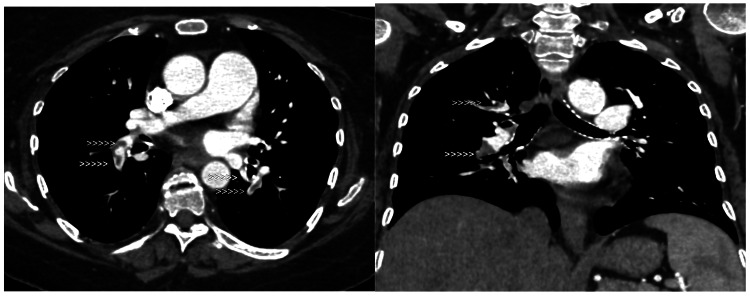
CT pulmonary angiogram showing acute pulmonary thromboembolism.
